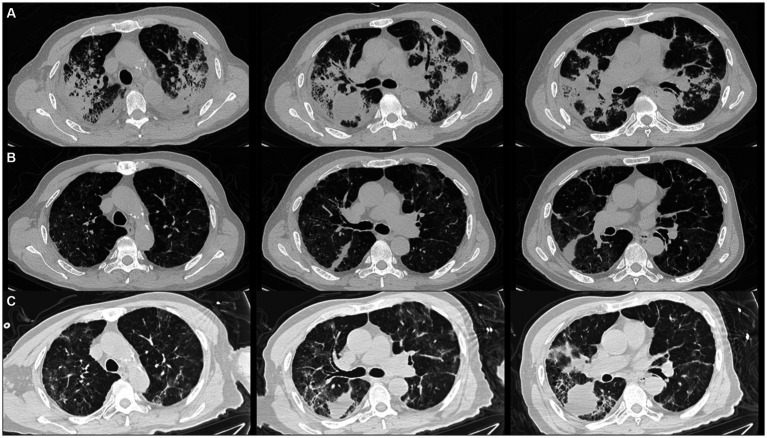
Patient’s chest computed tomography (CT) scan images at three time points. (A) Initial chest CT showed bilateral peribronchchial distribution of multifocal opacities and consolidations. (B) After taking methylprednisolone for 2 months, chest CT revealed that the bilateral opacities and consolidations were significantly absorbed. (C) After taking methylprednisolone for 3 months and the patient just stopped using it, chest imaging revealed that the bilateral opacities and consolidations were aggravated again.
